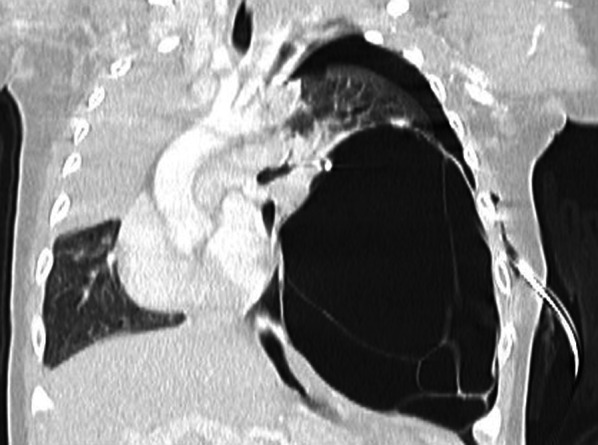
Contrast-enhanced computed tomography of the chest after insertion of a chest drainage tube. Severe mediastinal deviation to the right is evident, due to giant cystic lesion and pneumothorax
